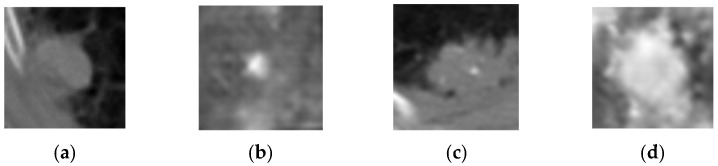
CT cases: (a) real benign, (b) generated benign, (c) real malignant, (d) generated malignant.
